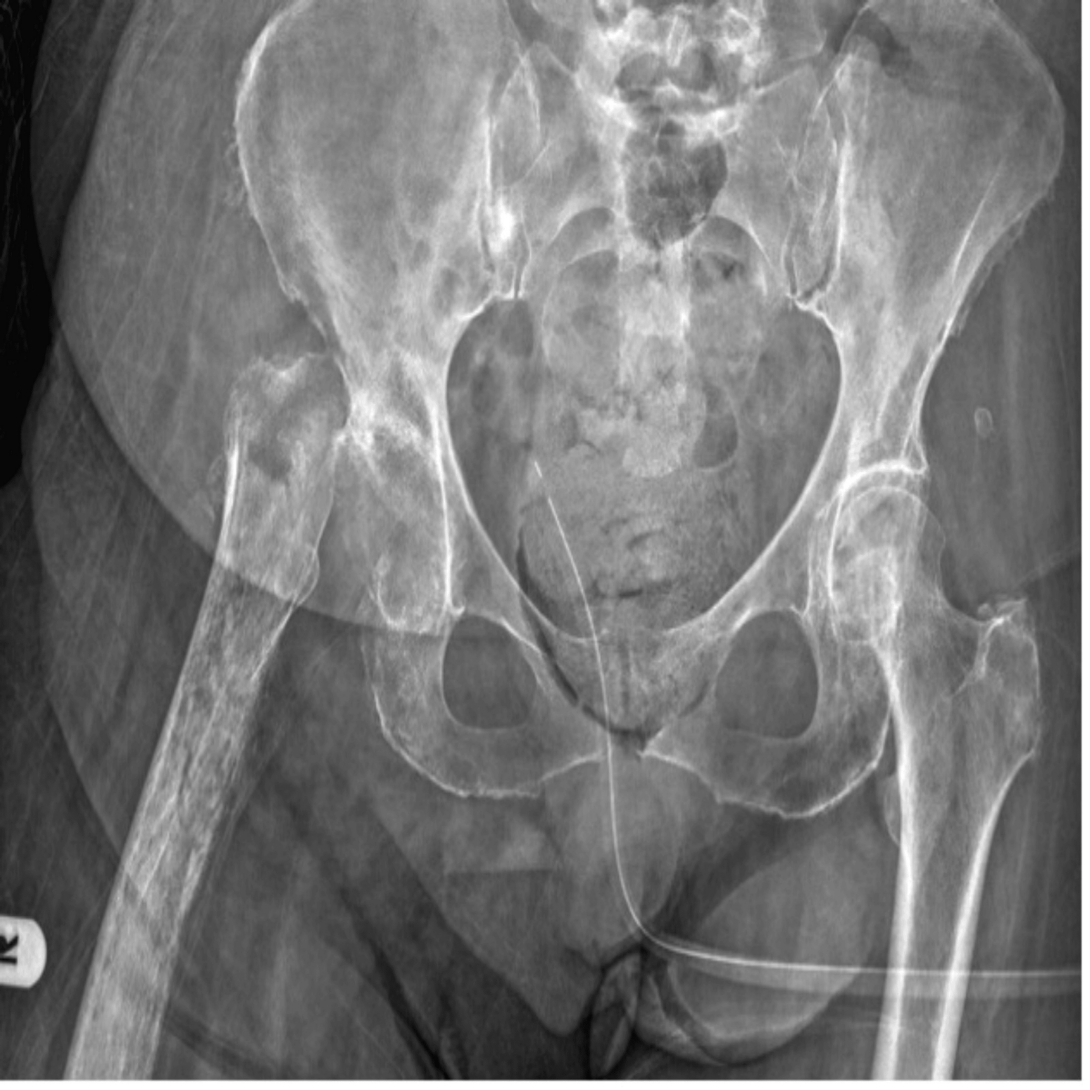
Plain radiograph before final revision surgery
